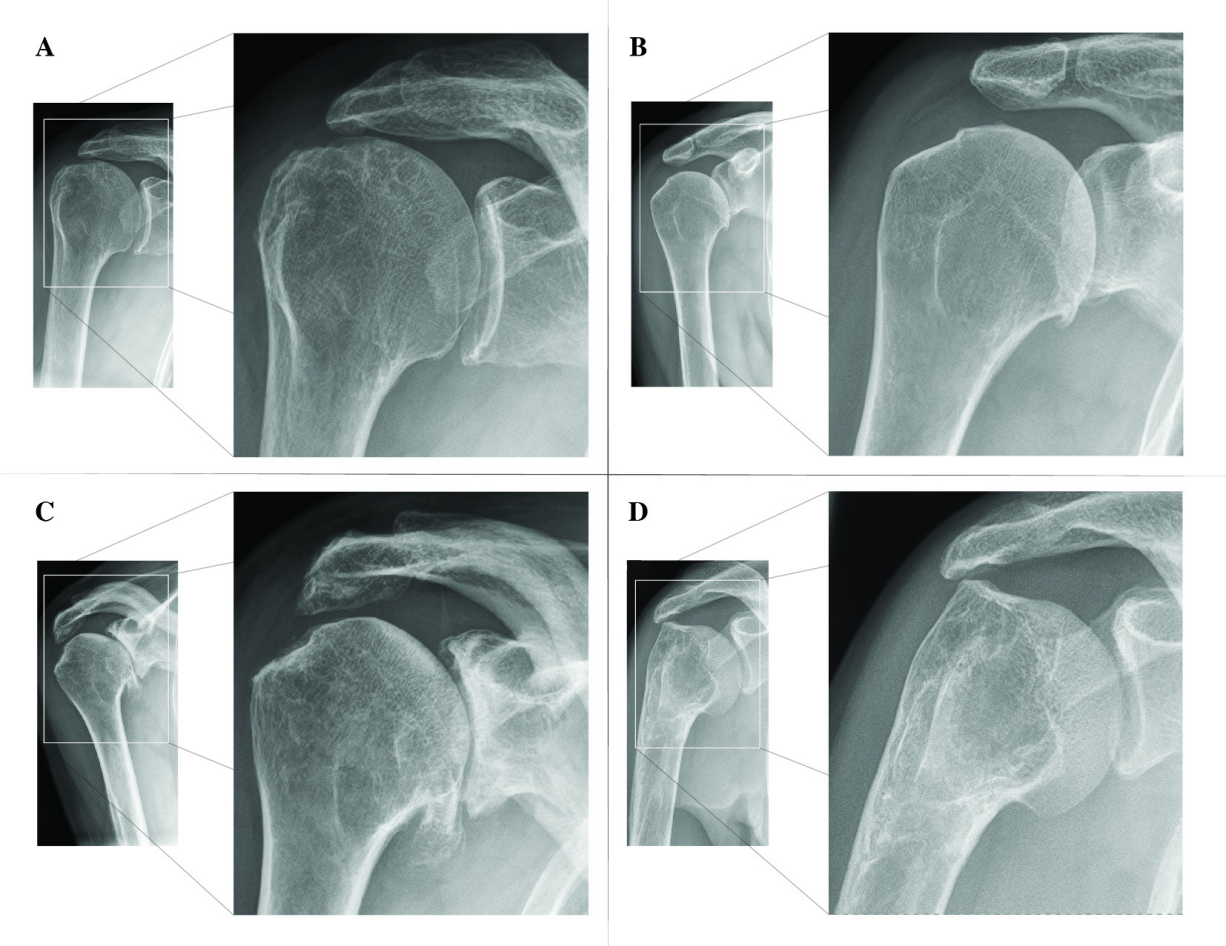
Examples of shoulder radiographs where dose was reduced to 84% (A), 71% (B), 60% (C) and 50% (D) of baseline. Bony cortex, trabeculae, joint spaces, overall contrast and soft tissue visualization were assessed on a five-point Likert scale
